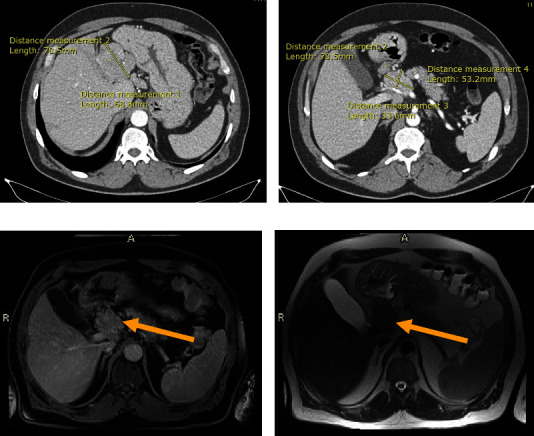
(a) Enhanced CT scan transversal view showing 6.8 × 5.1 left liver lobe mass. (b) Enhanced CT scan transversal view showing 5.3 × 3.3 lesion encasing the hepatic artery without obstructing it. (c) MRI showing 45 × 47 mm multinodular lesion hypo T1 diffusion restricting the lesion at the porta hepatis (yellow Arrow). (d) MRI showing 45 × 47 mm multinodular lesion hyper T2 diffusion restricting the lesion at the porta hepatis (yellow Arrow).
